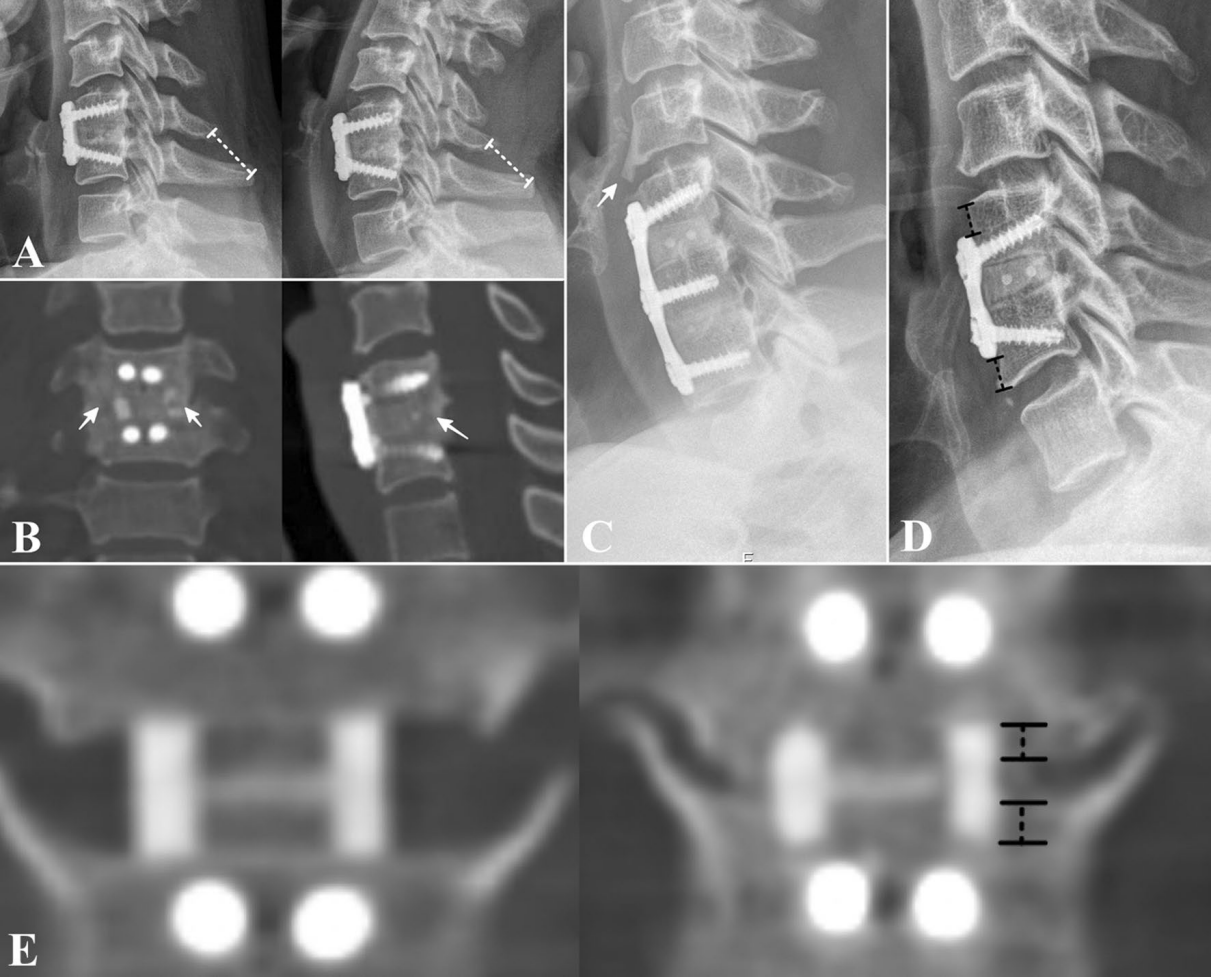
Radiographic measurements (A) Fusion by interspinous motion. Interspinous motion < 2 mm on 150% magnified flexion/extension lateral radiograph was considered as fusion. (B) Fusion was achieved by bone bridging. Bone bridging formation demonstrated both on coronal and sagittal reconstructed CT images were assessed as fusion. (C) Ossification of the anterior longitudinal ligament crossing the adjacent disc space demonstrated on lateral cervical radiograph was considered as an adjacent level ossification development. (D) Plate-adjacent disc space distance. The distance between the tips of the plate to the cephalad and caudal adjacent disc spaces on the immediate postoperative lateral radiograph of the cervical spine is shown. (E) Assessment of subsidence. Amount of subsidence was measured by comparing the distance between endplate of vertebral body and edge of the allograft (dotted lines) measured at CT taken 2-days and 1 year postoperatively. Subsidence of > 2 mm demonstrated in at least one of upper or lower endplate-allograft interface was defined as significant subsidence.
